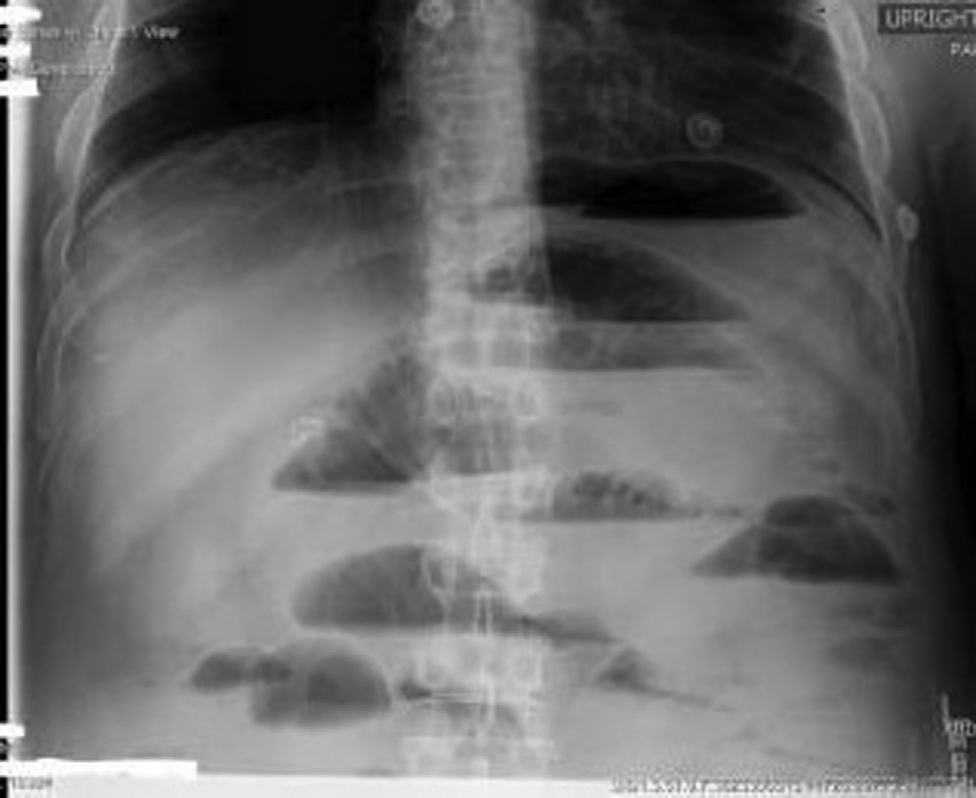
Erect abdominal radiograph showing multiple air fluid levels.
